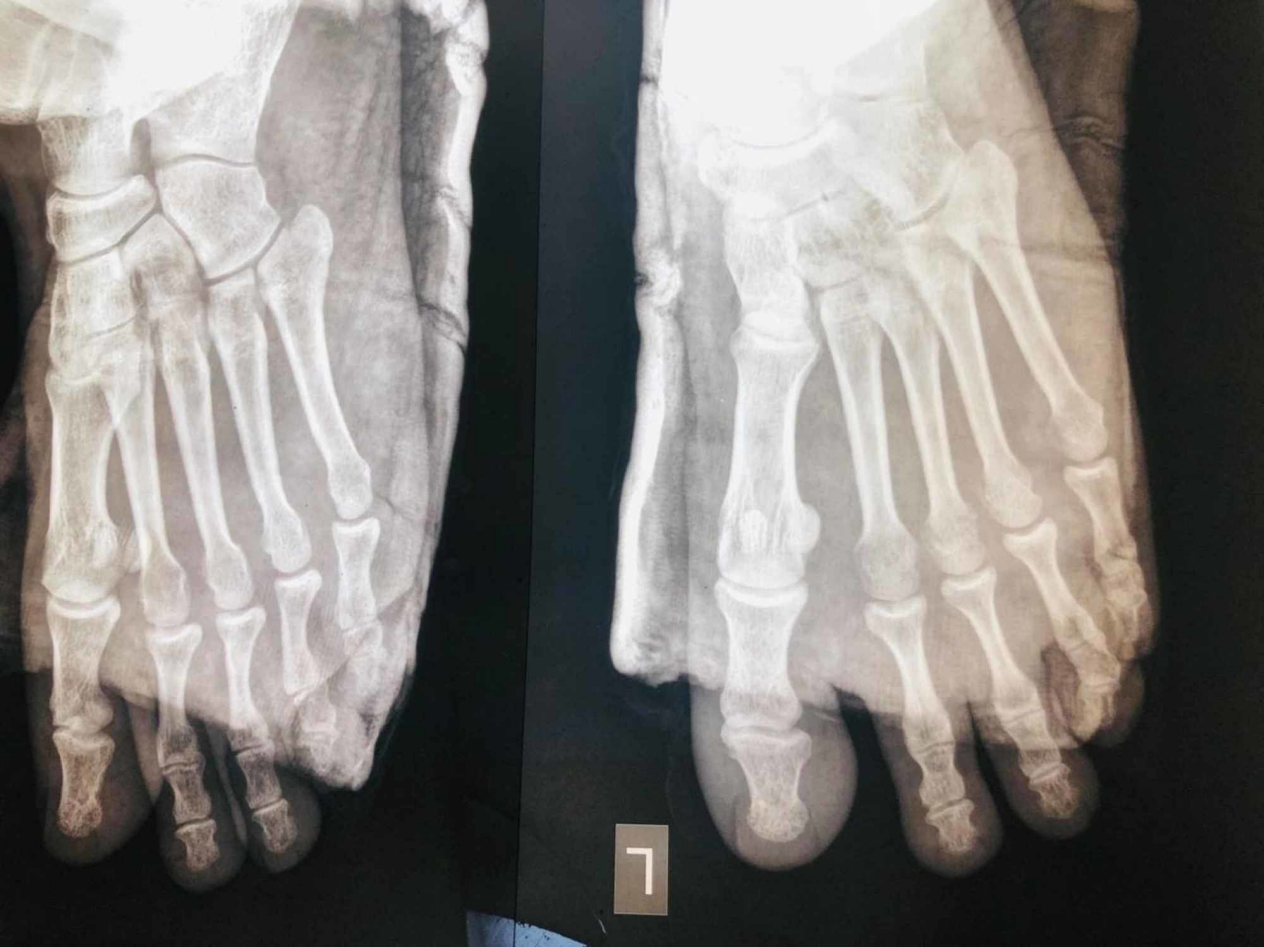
Immediate post operative X-ray shows stable reduction of all metatarsophalangeal joints.
